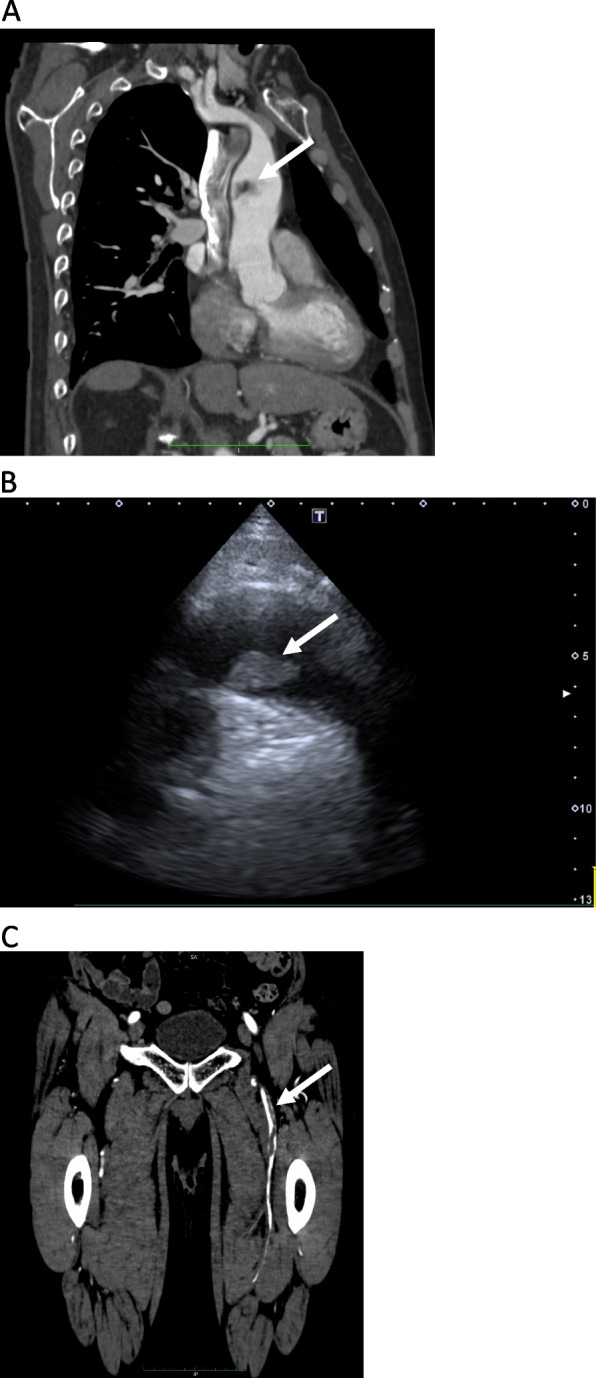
A computed tomography angiography (A) and a transthoracic echocardiography (B) demonstrated that a floating mass measuring 23 × 14 mm and 26 × 13 mm was located at ascending aortic lumen and an embolism in the left superficial femoral artery (C)
